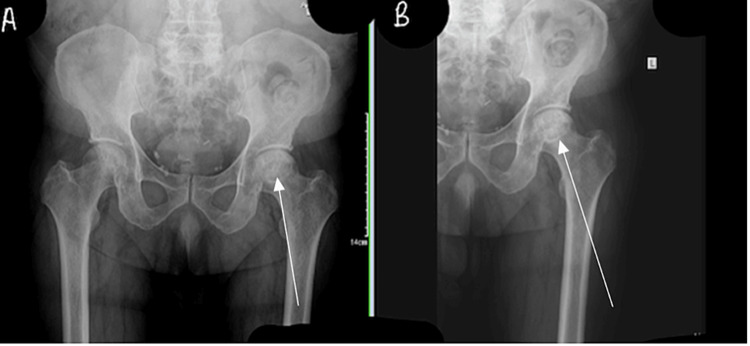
Anteroposterior radiograph of the pelvis (A) and left hip (B). Arrows show stage II avascular necrosis of the left hip without collapse of the left hip.
